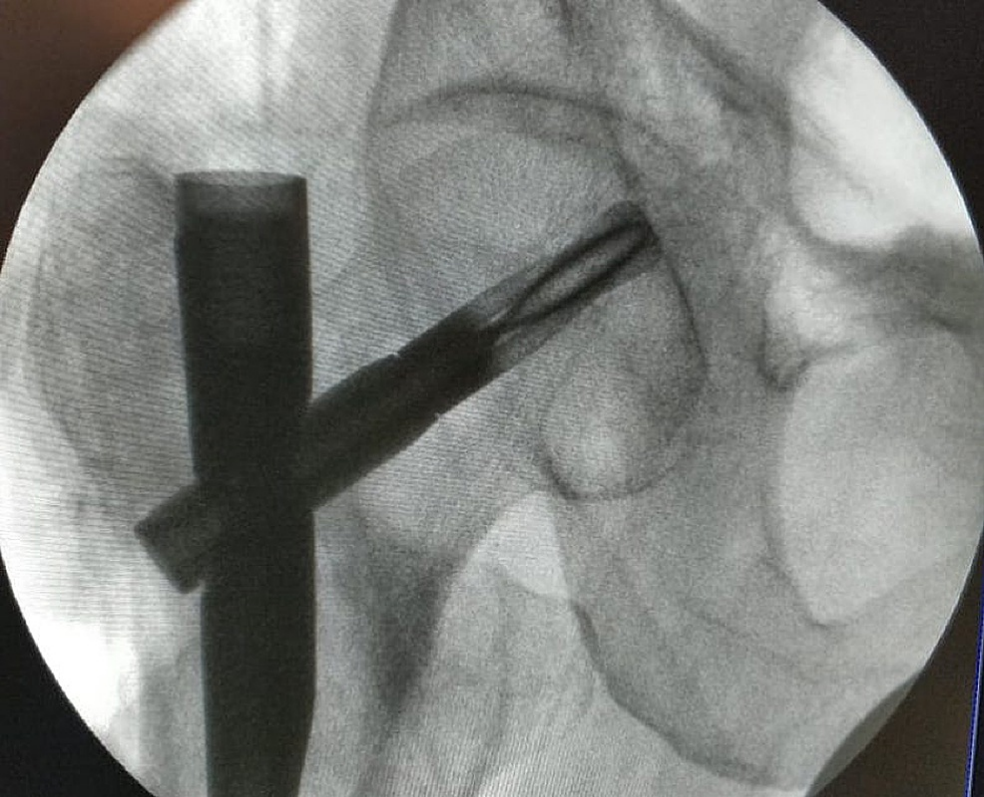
Final radiograph with nail and the new blade in situ
